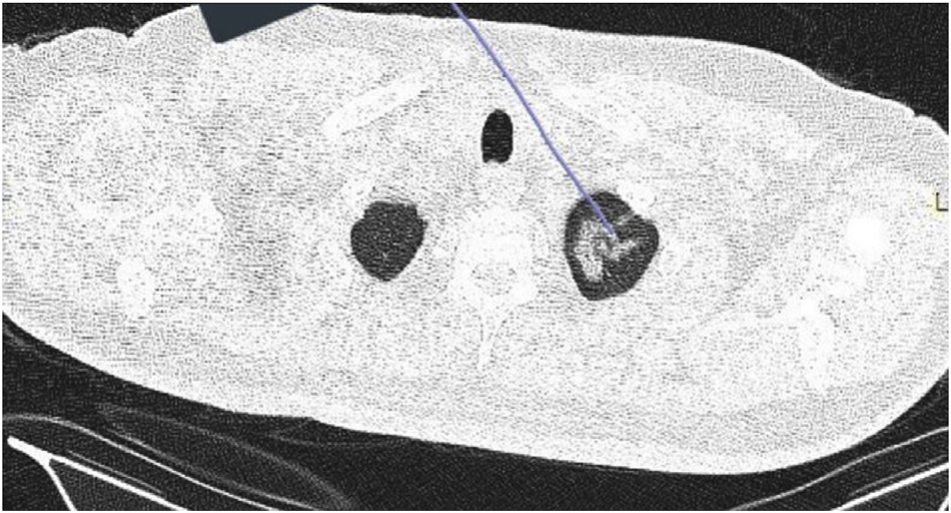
Chest CT-scan (axial section) of the second case showing bilateral diffuse ground glass opacities with consolidation.
